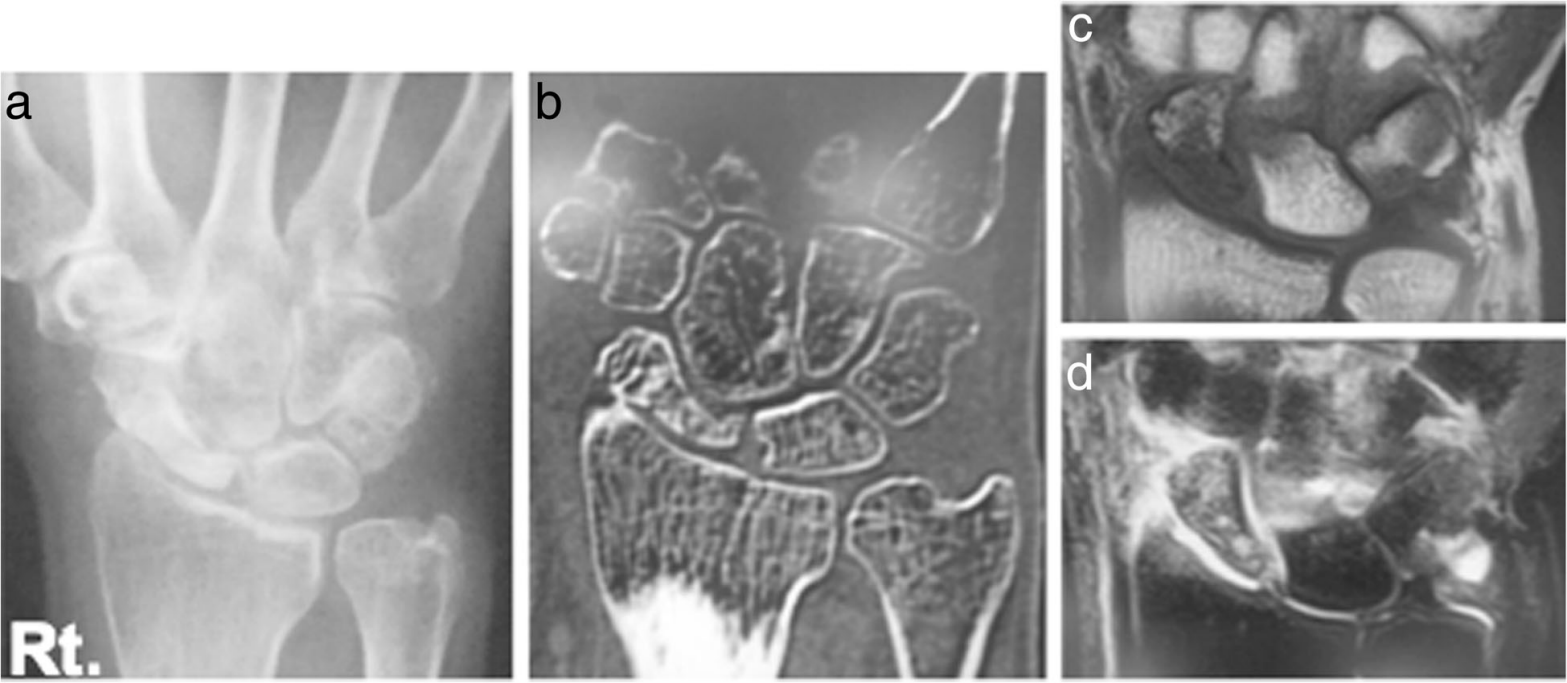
Radiograph (a), and magnetic resonance images (b, c, d). Sixty-one-year-old female patient with scaphoid nonunion advanced collapse stage III. No previous trauma was noted
